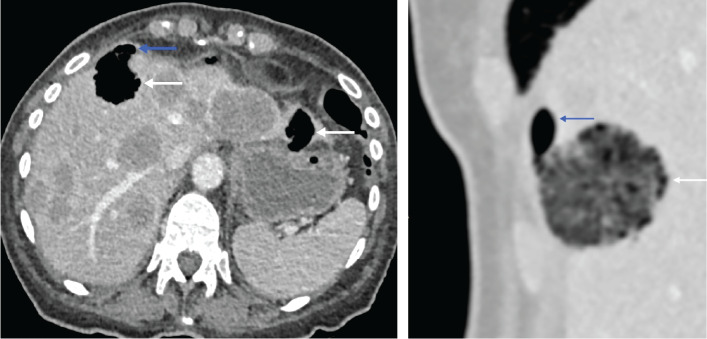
Follow-up CT scan (July 11, 2025) showing gas bubbles in several hepatic metastases (white arrows) and minimal pneumoperitoneum (blue arrows) in axial and sagittal planes.
